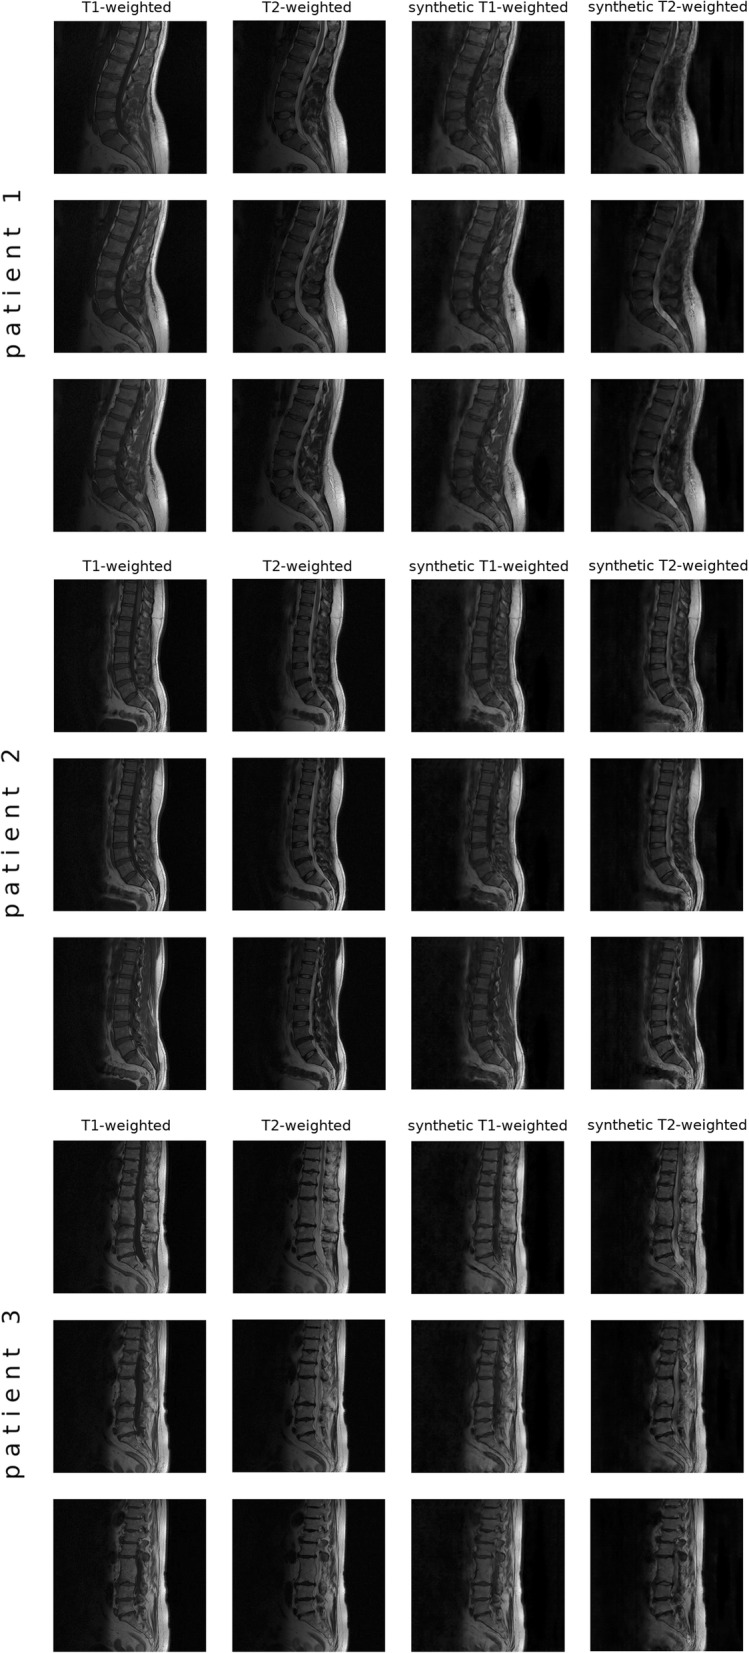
Representative results (three slices for three different patients) of the translation from T1W to T2W MRI and vice versa. In the first patient, Type II Modic changes were correctly represented in both synthetic images, whereas for the Type I change in the third patient the synthetic T2W image showed a low signal instead of a high one. The L4-L5 disc protrusion of the second patient was accurately represented in the synthetic T2W image
